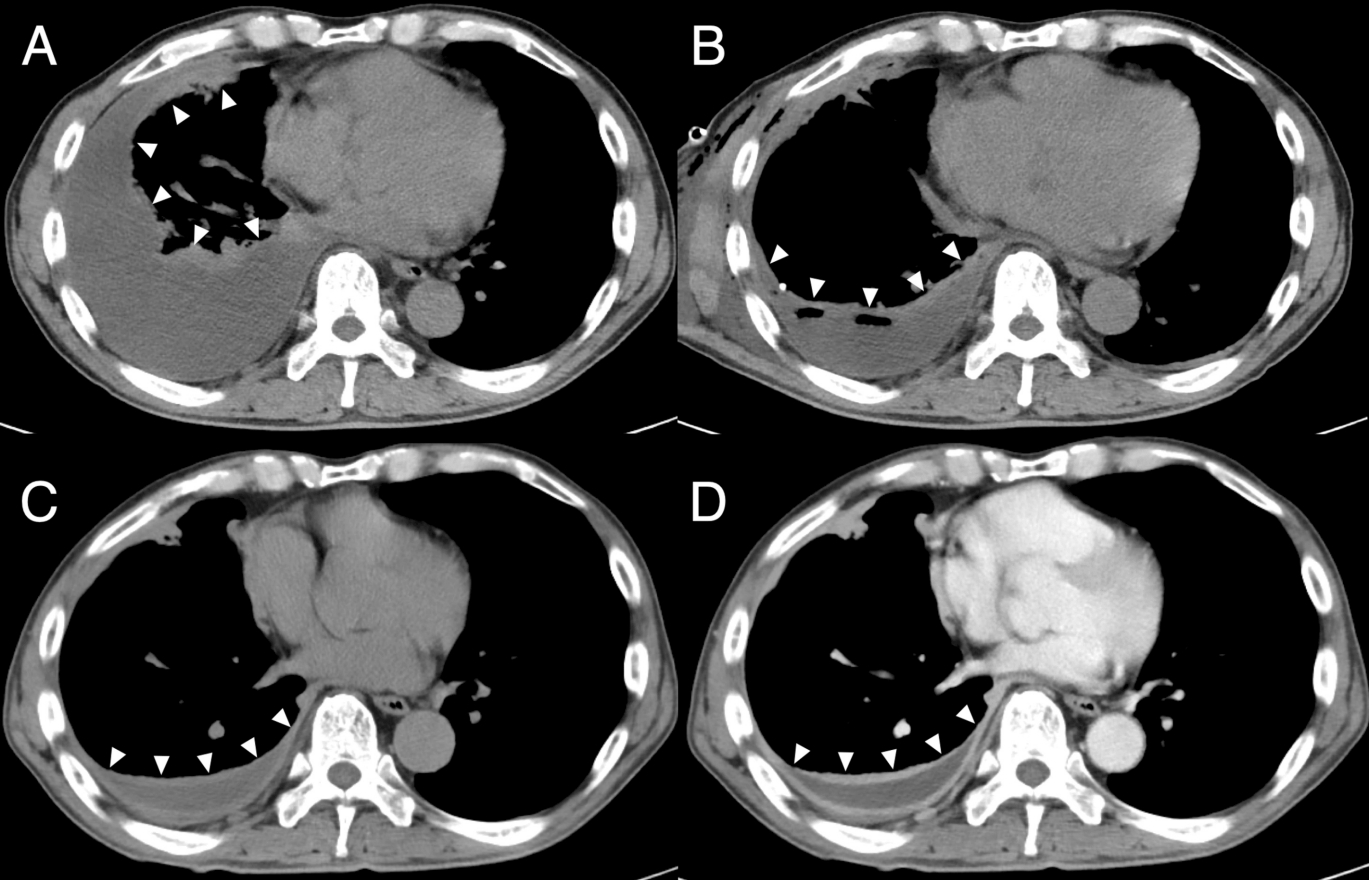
(A) CT scan on admission (seven days before the initiation of anti-tuberculosis therapy) showing right pleural effusion (white arrowheads). (B) CT scan on the second day of admission (five days before the initiation of anti-tuberculosis therapy) after pleural drainage, showing a reduction in effusion volume (white arrowheads). (C, D) CT scan on the 34th day of admission (27 days after the initiation of anti-tuberculosis therapy) (C: non-contrast, D: contrast) showing further improvement in right pleural effusion (white arrowheads).
